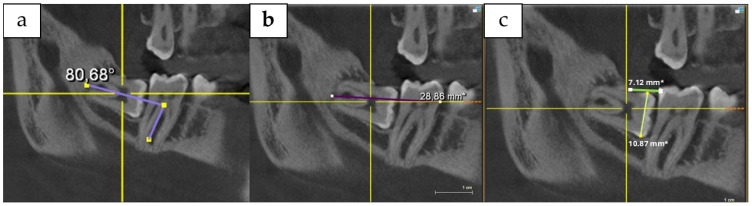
The difficulty index of the surgery was calculated by evaluating (a) spatial relationship, (b) depth, and (c) ramus relationship/space of third molar. In this method, the DI of the lower third molar extraction is measured using CBCT, based on its spatial relationship, depth, and ramus relationship/space. Each tooth is graded in each aspect, and the sum of these grades places the tooth into one of four categories: DI I to DI IV.
